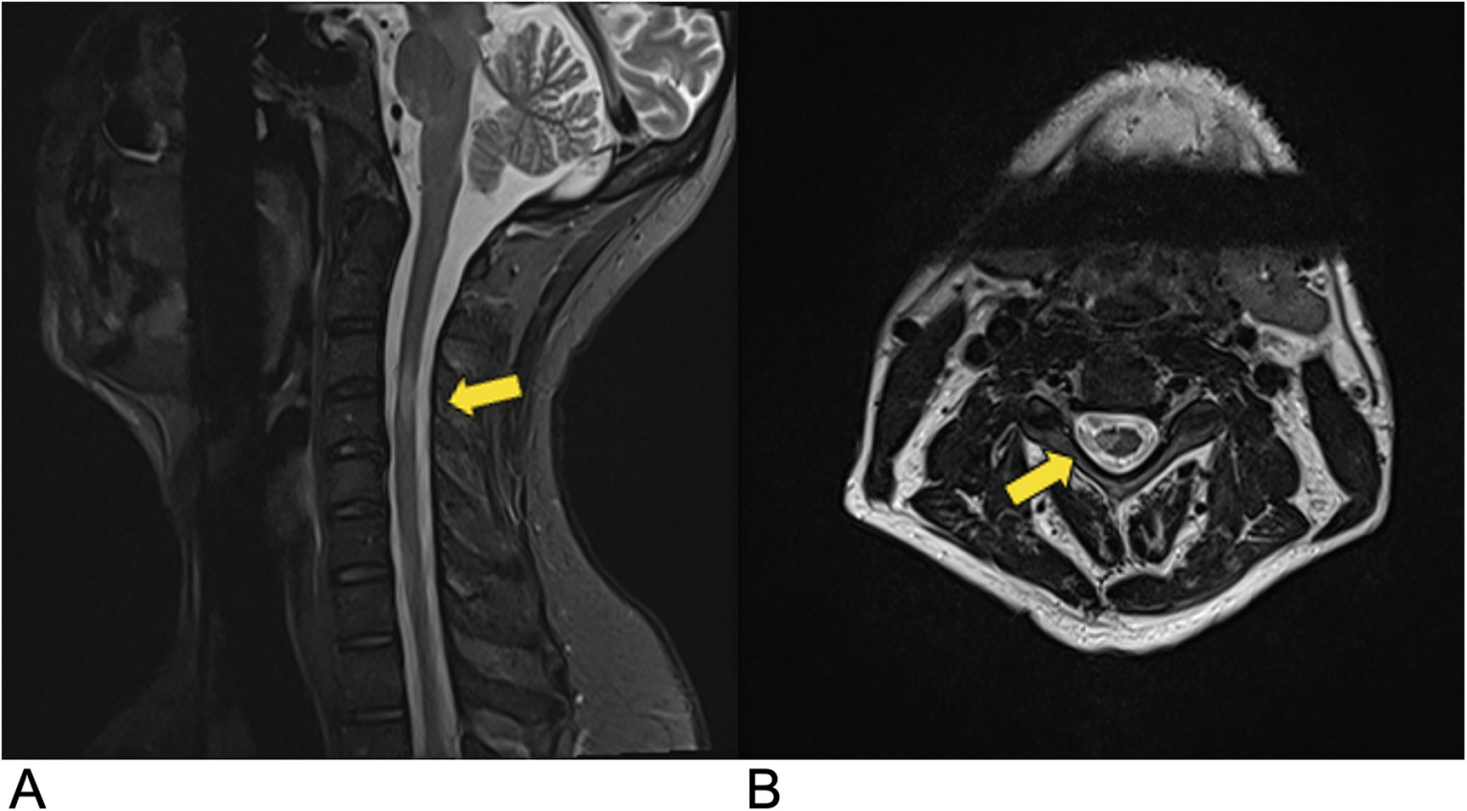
Demyelinating disease. Sagittal (A) and axial (B) T2-weighted MRI demonstrating multiple demyelinating plaques in the spinal cord (yellow arrows) consistent with multiple sclerosis.
